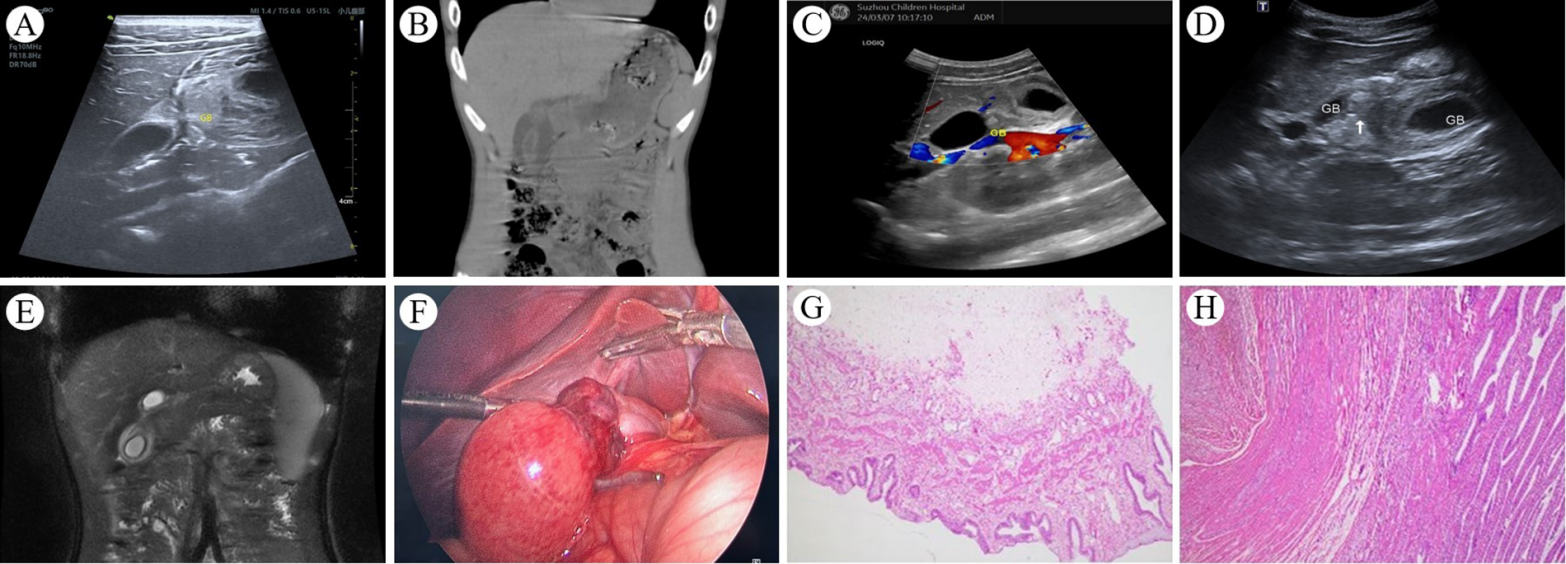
(A) Upon the child's first hospital admission, an abdominal ultrasound revealed a linear hypoechoic area in the body of the gallbladder, with thickening and edema of the walls at the body and base; (B) An abdominal CT scan showed a rough and thickened gallbladder wall, fluid accumulation in the gallbladder fossa, and a small amount of pelvic effusion; (C) abdominal ultrasound demonstrated a linear hypoechoic area in the body, with significant thickening of the walls at the body and base, and blood flow signals were detectable within the hypoechoic region. (D) During the child's second hospital admission, abdominal ultrasound showed a marked enlargement of the base and body of the gallbladder, with pronounced thickening of the walls, and a whirlpool-like hyperechoic area was observed between them; (E) Abdominal MRI combined with MRCP indicated an enlarged gallbladder, with a “figure of eight sign” at the middle part of the body, the gallbladder wall was significantly thickened and rough, and fluid was visible in the gallbladder fossa; (F) Intraoperative findings revealed a markedly enlarged gallbladder base, with significant edema and thickening of the wall, and torsion of the body. (G and H) chronic cholecystitis associated with GT.
